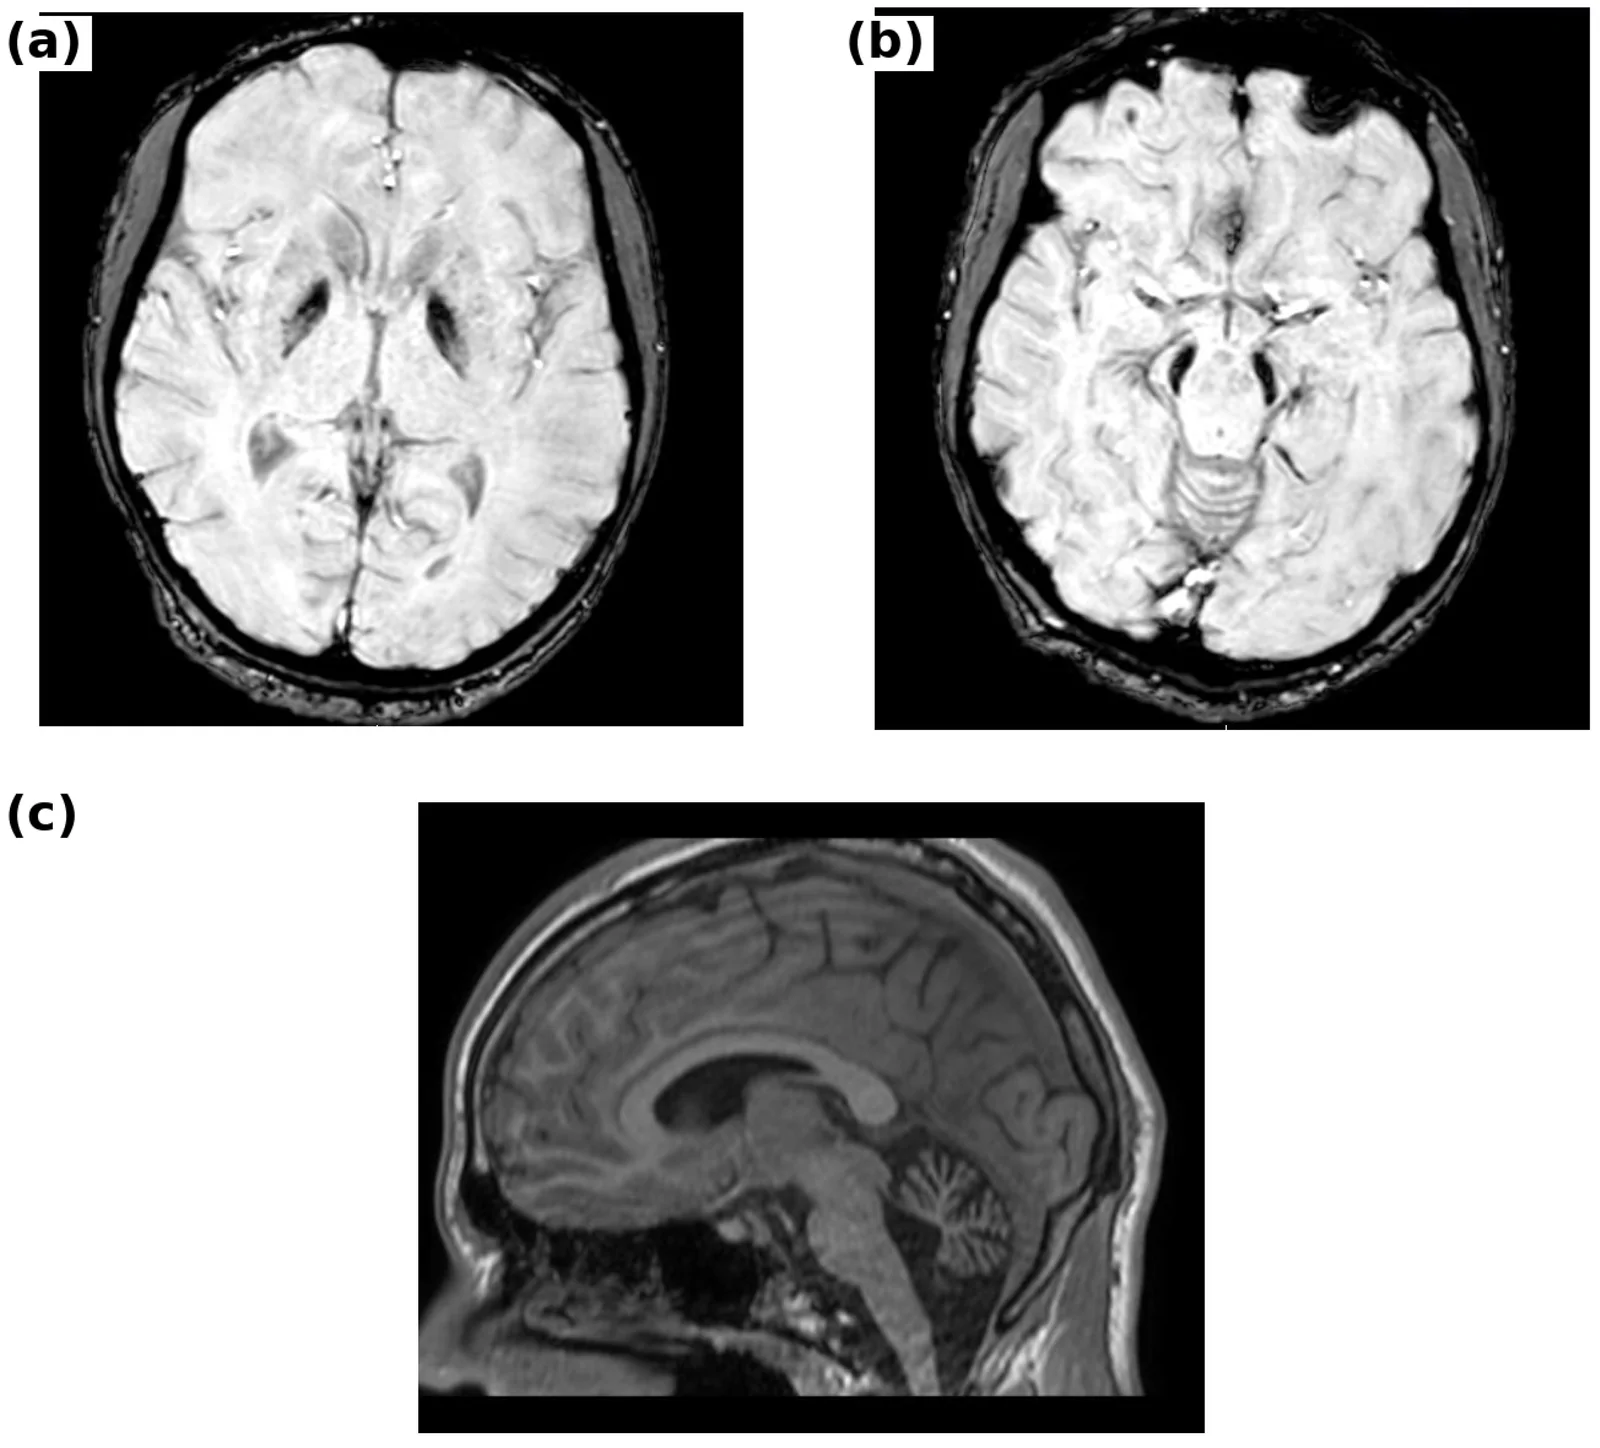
Brain MRI of Patient 1. (a) Axial susceptibility-weighted image showing bilateral hypointensity of the globus pallidus. (b) Axial susceptibility-weighted image showing hypointensity of the substantia nigra. (c) Sagittal T1-weighted image showing marked cerebellar atrophy.
